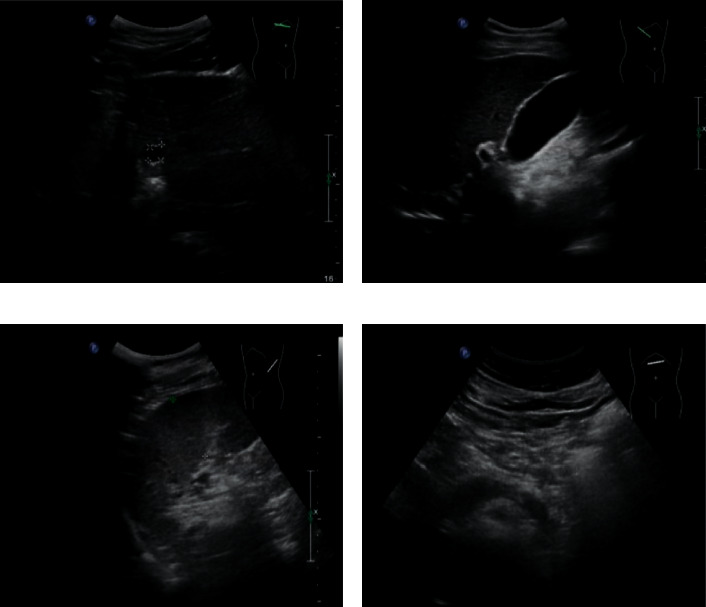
The picture of contrast-enhanced ultrasound for liver cancer at stage I.
